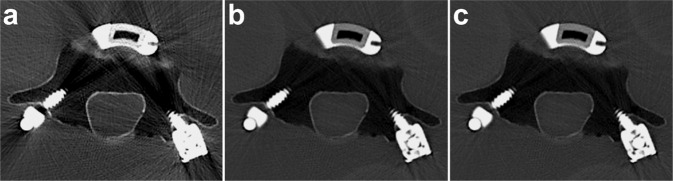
High energy virtual monochromatic image (VMI) reconstructions allow for artifact reduction. Axial (a) 70 keV, (b) 100 keV, and (c) 140 keV VMIs reconstructed from the same dual-energy CT (DECT) scan are shown. The images are displayed using the same window-level display settings to enable a direct and unbiased comparison. Note the significant reduction in artifact and image noise on the high energy VMIs at 100 keV (b) or 140 keV (c) compared to the 70 keV VMI (a), which is typically considered to correspond to the conventional single energy CT scan. Of note, the images shown here are the qualitative correlates of the attenuation measurements shown in Fig. 3
